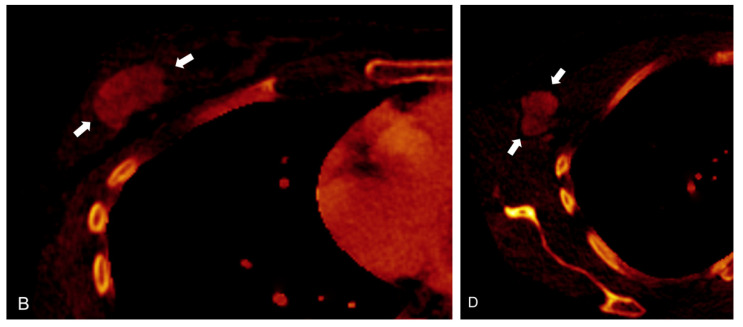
Axial contrast-enhanced dual-energy CT (DECT) scan of a 40-year-old patient with grade 2 luminal B invasive ductal carcinoma in the right breast. Linearly blended M_0.6 image series show a lesion in the right breast (A), as well as enlarged, round lymph nodes in the right axilla (B). In the present study, DECT iodine map radiomic signatures derived from the primary tumor (C) yield a mean AUC of 0.82 for separation of metastatic and non-metastatic breast cancers; in addition, substantial textural differences exist between primary tumor and metastatic tissue (D).
